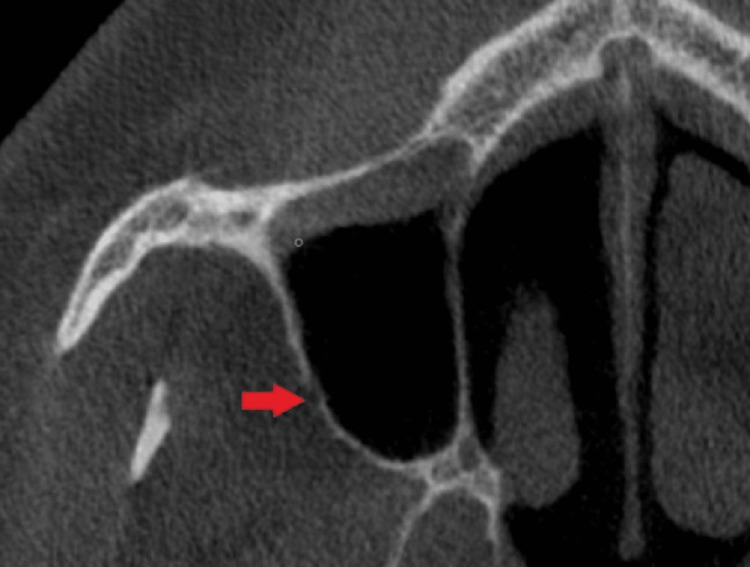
Diameter measurement of the AAA using CBCT. AAA = alveolar antral artery; CBCT = cone-beam computed tomography
